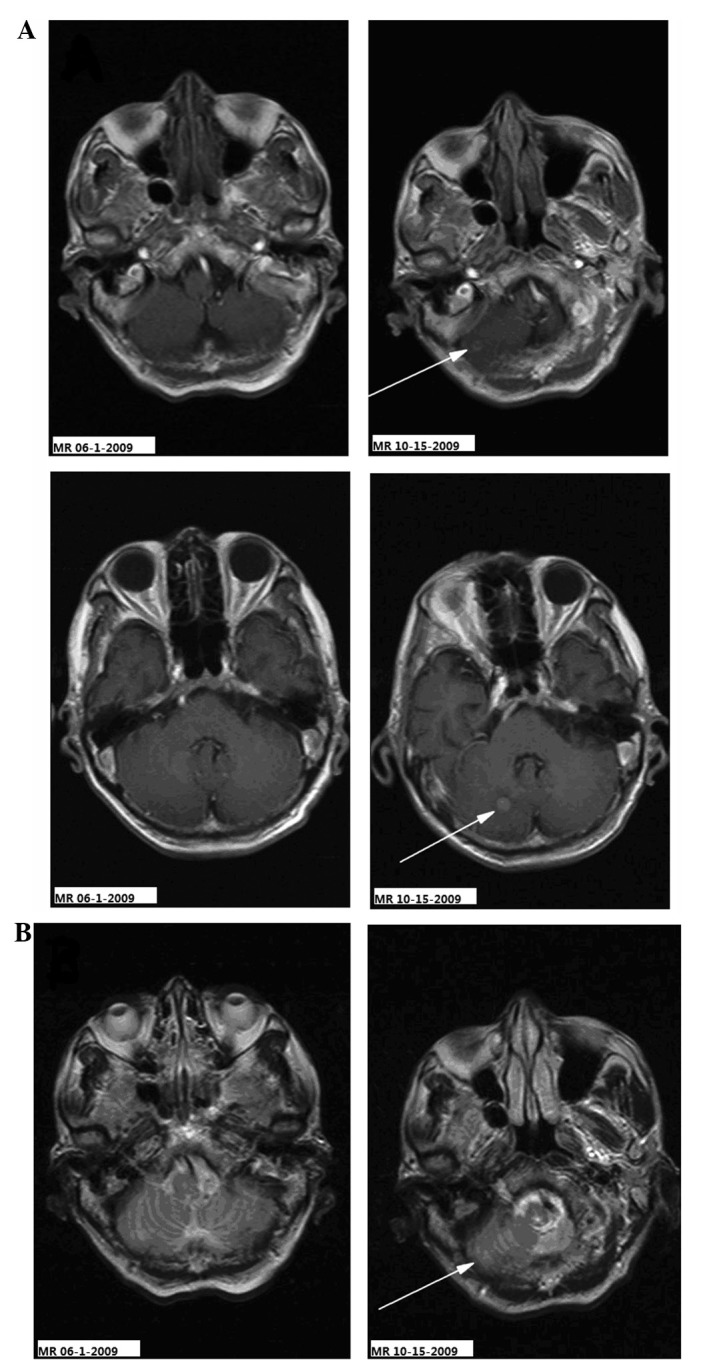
Comparison between the cerebral MR images of the esophageal cancer patient obtained prior to (MR 06-1-2009) and following (MR 10-15-2009) the metastasis. (A) Enhanced axial T1-weighted images and (B) axial T2-weighted images. Left (MR 06-1-2009), no abnormal signals were observed in the brain; and right (MR 10-15-2009), abnormal signals were observed in the right cerebellar hemisphere (indicated by the arrows). MR, magnetic resonance.
